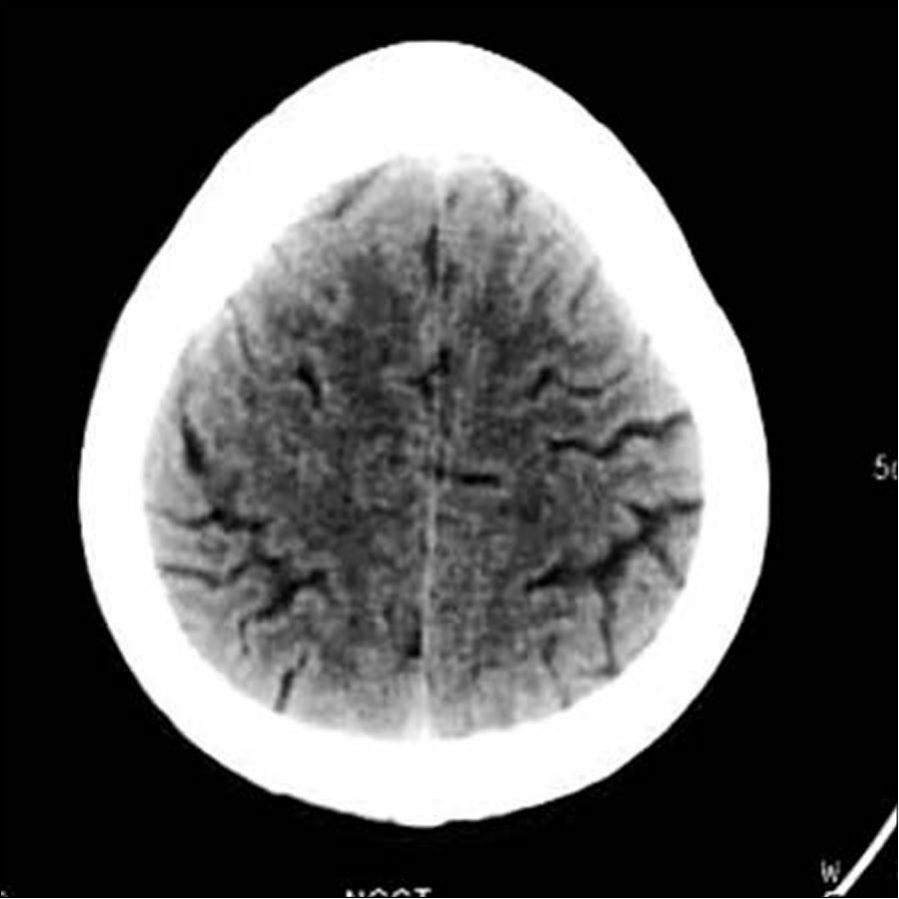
Axial CT scan of a patient not showing any focal lesion (study case Images taken 9 h after ischemic stroke)
